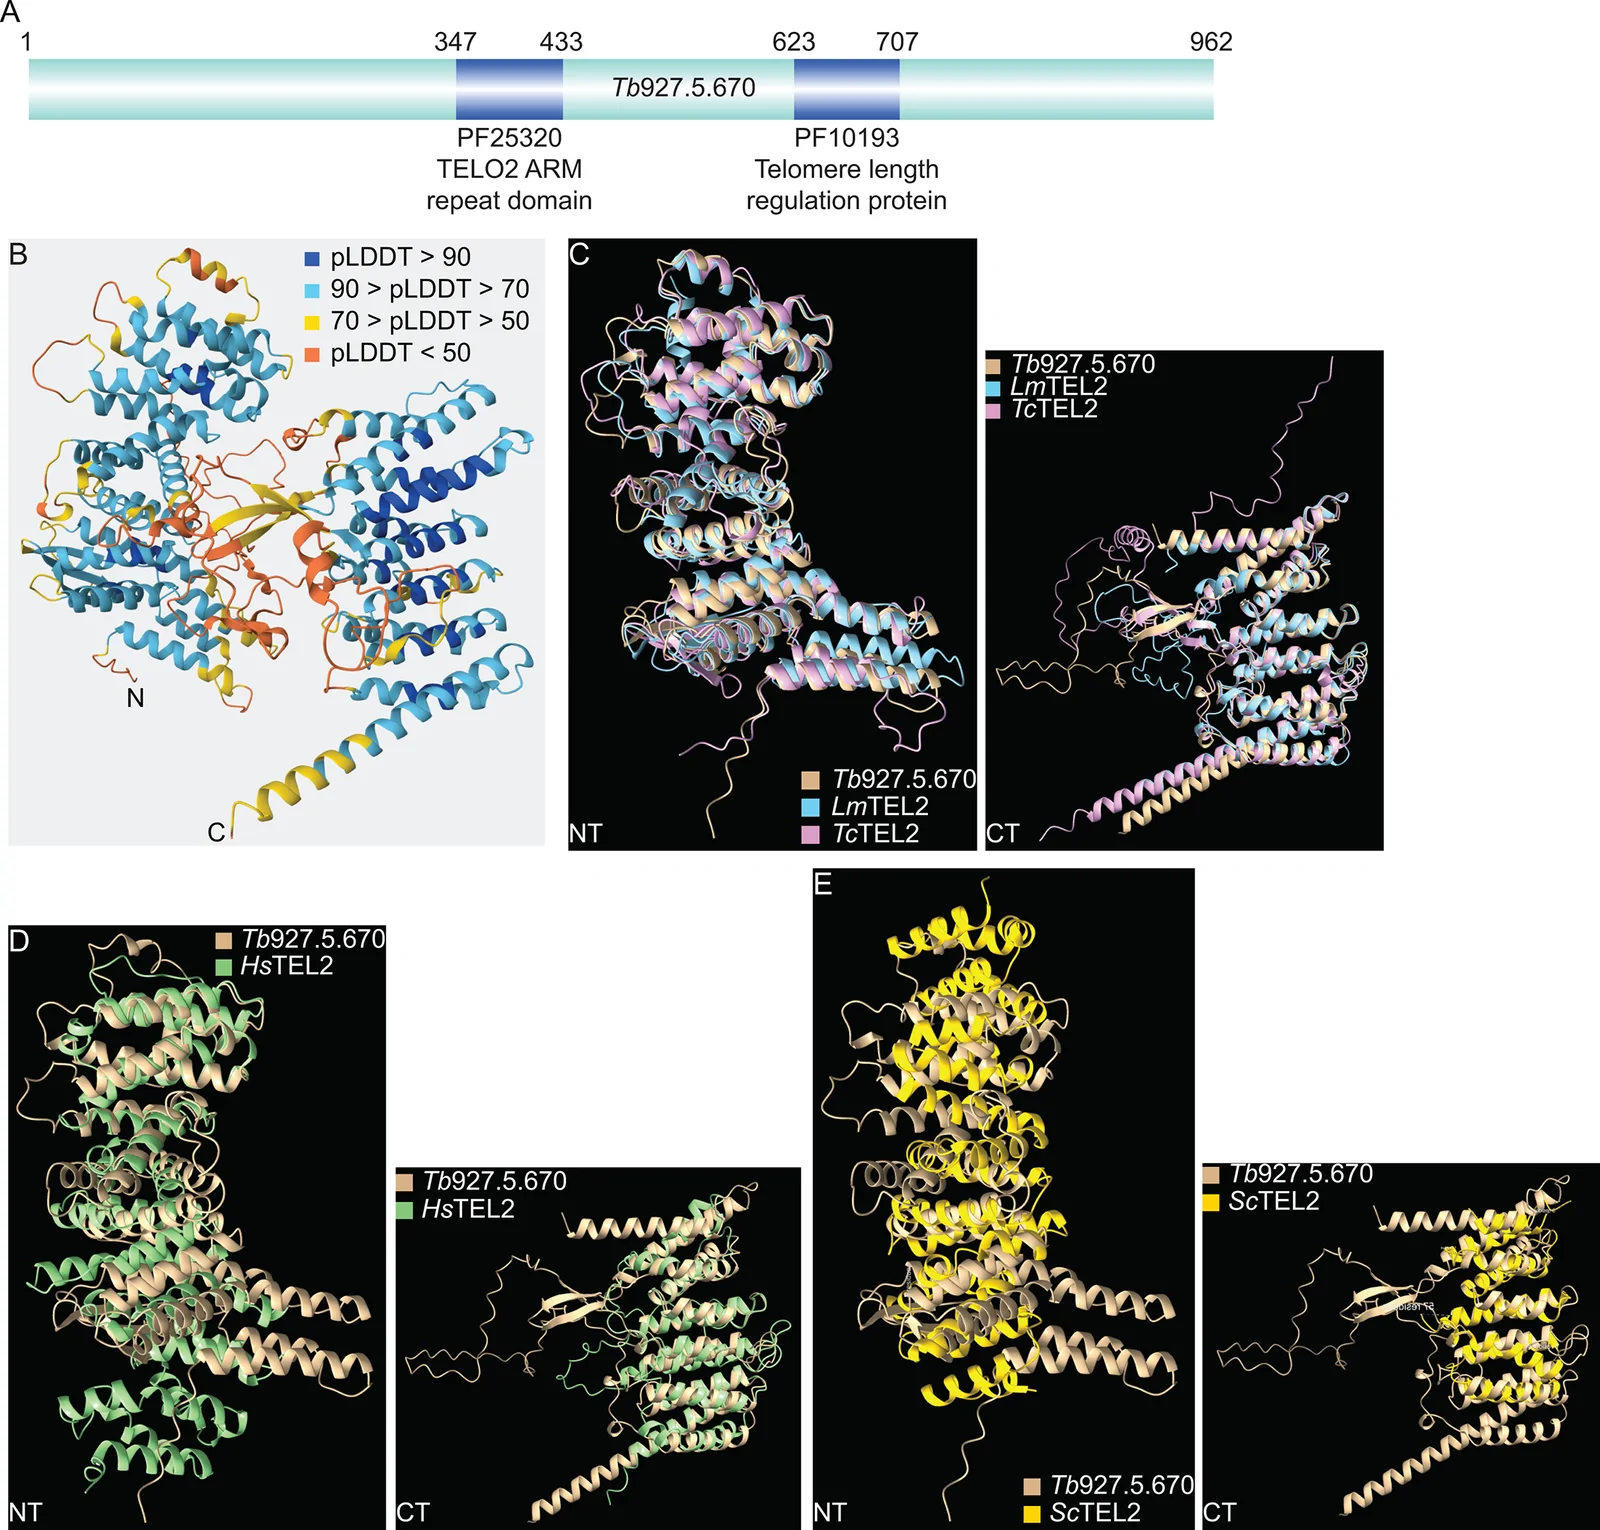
Tb*927.5.670 is a TEL2 homolog. (A) Domain structure of *Tb*927.5.670. PF25320, a predicted ARM-type repeat region in TEL2 homologs. (ARM repeats are α-helical repeat motifs that form elongated, superhelical solenoids specialized for protein–protein interactions.) PF10193, a conserved, TEL2-specific structural domain required for the TEL2-TTI1-TTI2 (TTT) scaffold that is needed for PIKK maturation. (B) AlphaFold 3-predicted protein structure of *Tb*927.5.670, which has an N-terminal (NT, left) and a C-terminal (CT, right) portion connected by flexible loops (middle). pLDDT: a per-atom confidence estimate on a 0–100 scale, where a higher value indicates higher confidence (61, 62). (C) Alignments of AlphaFold 3-predicted structures of *Tb*TEL2, *Lm*TEL2, and *Tc*TEL2 NT (left) and CT (right) halves using ChimeraX. (D) Alignments of AlphaFold 3-predicted structures of *Tb*TEL2 and *Hs*TEL2 NT (left) and CT (right) halves using ChimeraX. (E) Alignments of *Tb*TEL2 NT (left) and CT (right) structures (AlphaFold 3-predicted) and corresponding regions of *Sc*TEL2 (solved X-ray structure, PDB ID: 3O4Z [51]) using ChimeraX. Additional alignment parameters for panels C–E are listed in Table S2.
